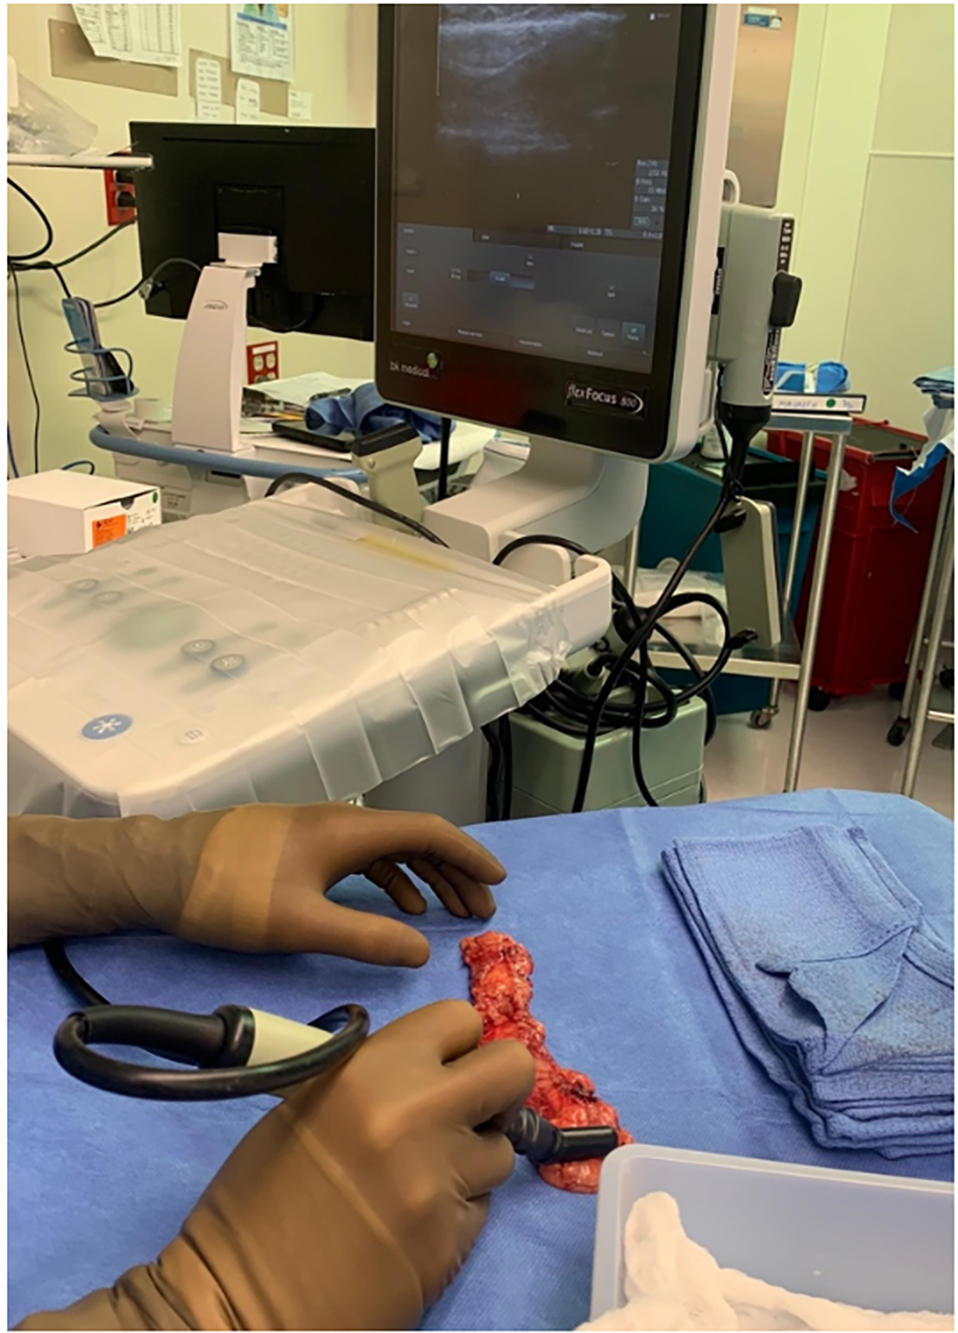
Intraoperative duplex ultrasonography of the gastroepiploic omental lymph node flap for the quantification of transferred lymph nodes.
